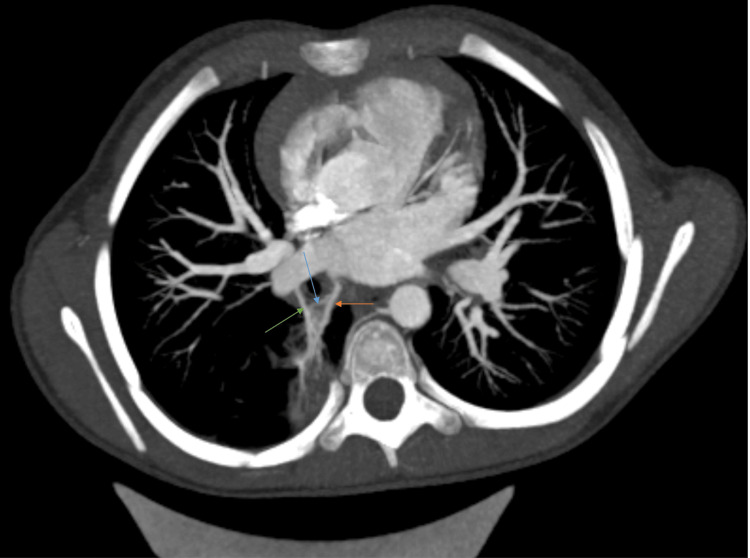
Chest CT angiography (mediastinal window) in axial plane with maximum intensity projection All three branches together: blue arrow, arterial supply from the descending aorta; green arrow, branch coming from the right inferior pulmonary vein; orange arrow, anomalous venous drainage channel draining directly into the left atrium.
